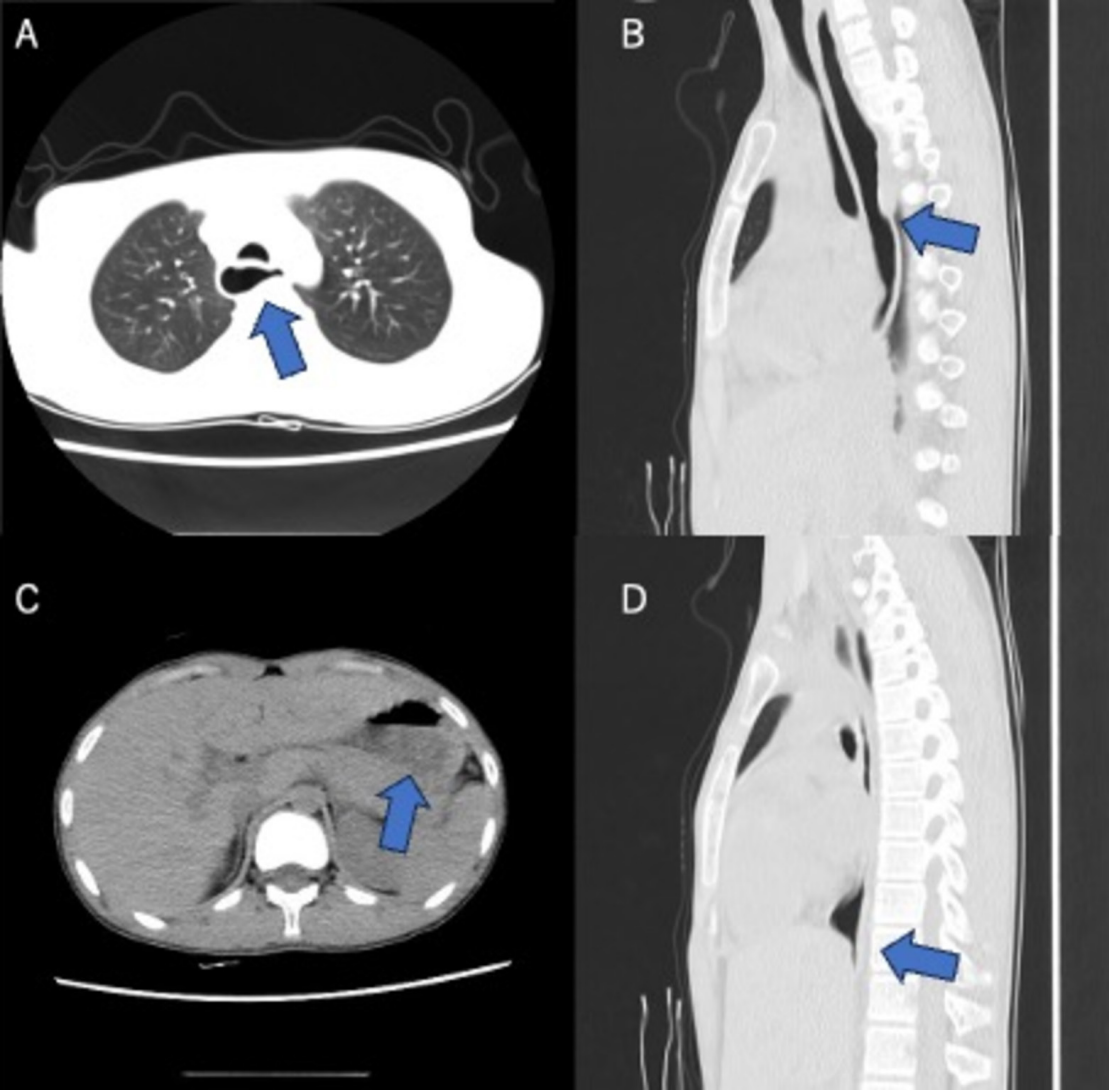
(A–D) CT imaging demonstrates persistent oesophageal dilation filled with air, without food retention or distal tapering, in a patient with rumination syndrome. (A) Axial view of upper oesophagus. (B) Sagittal view shows dilation along the thoracic spine. (C) Food residue that has passed through the oesophagus is observed in the stomach. (D) Sagittal image showing open gastroesophageal junction with no tapering.
